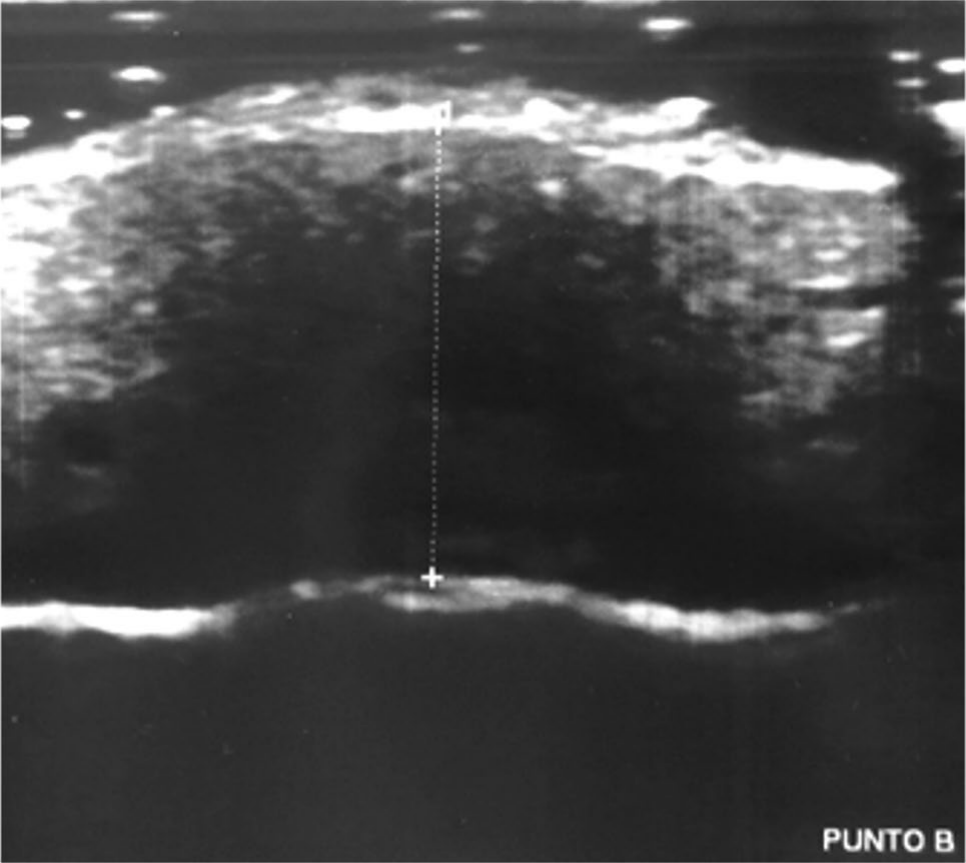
Ultrasonographic soft tissue thickness over B cephalometric point
